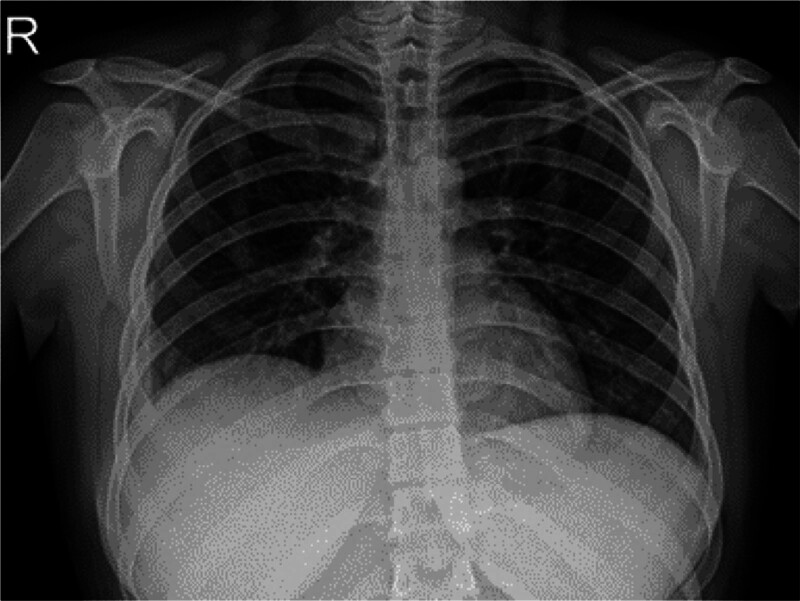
Normal appearance of Chest XRay in patient #3.
